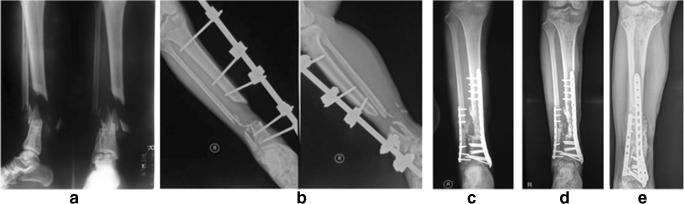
A 33-year-old male (case 5) with 6-cm bone defect of the tibia. a Initial radiography. b Pre-operative radiography. c Post-operative radiography. d Six-month post-operative radiography. e Twelve-month post-operative radiography
